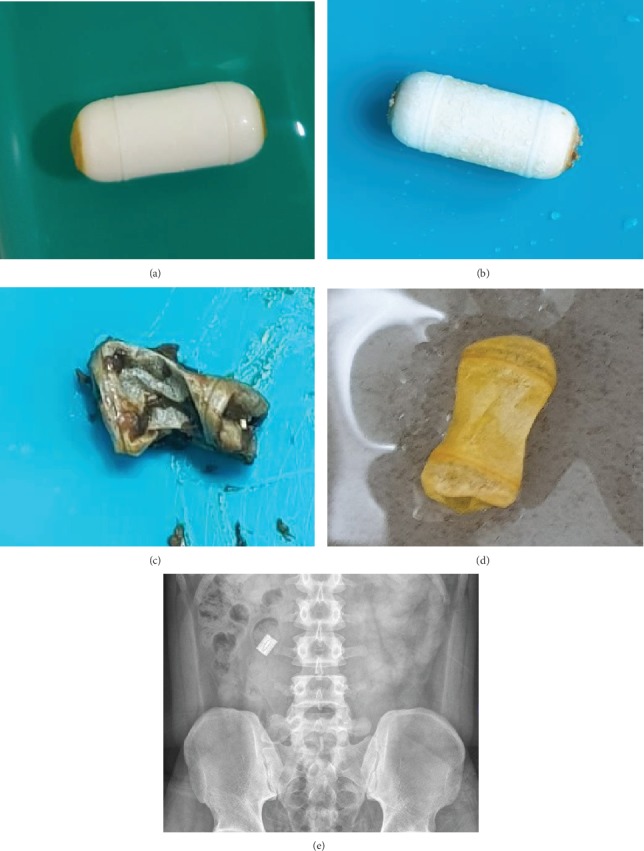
Identification of passed patency capsule: (a, b) intact and (c, d) disintegrated. (e) Patency capsule is observed on an abdominal X-ray image.
